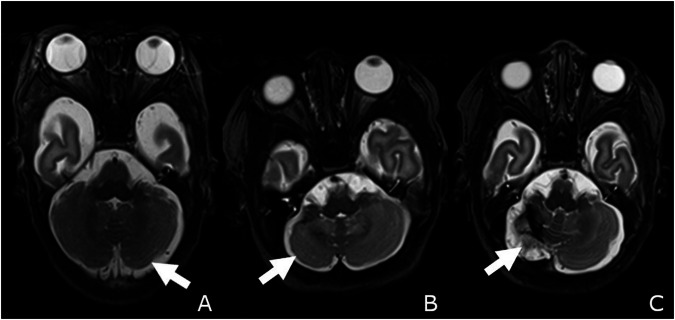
Cerebellar hypoplasia: Mild (1°, white arrow in A), moderate (2°, white arrow in B), and severe (3°, white arrow in C) cerebellar hypoplasia. A Male infant born at 26 weeks of gestation with mild cerebellar hypoplasia on the left side (1°, < 25% hypoplasia of the affected cerebellar hemisphere). B Male infant born at 25 weeks of gestation and moderate hypoplasia on the right side (2°, 25–50% hypoplasia of the affected cerebellar hemisphere). C Female infant born at 28 weeks of gestation with severe hypoplasia on the right side (3°, > 50% hypoplasia of the affected cerebellar hemisphere, all T2-weighted TSE-sequences)
